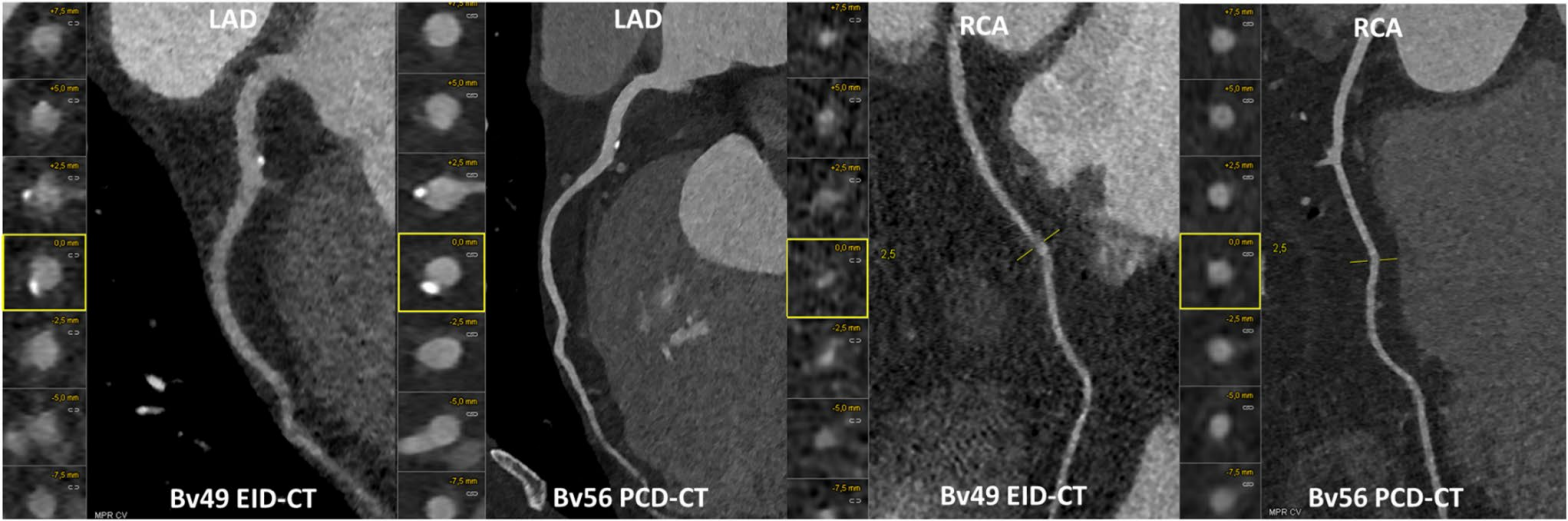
Multiplanar reconstructions of the coronary arteries of a 65-year-old post-heart transplantation patient using energy-integrating detector CT and photon-counting detector CT. On energy-integrating detector CT (EID-CT), the proximal segment of the left anterior descending artery displays a small calcification with an image quality score of 4-very good. On photon-counting detector CT (PCD-CT), the calcification is characterized as a mixed plaque (non-calcified plaque surrounding the calcification) with an image quality score of 5-excellent. For the right coronary artery, the entire artery was scored as 5-excellent with no plaque on PCD-CT. On EID-CT, the proximal right coronary artery segment was scored as 3-good with non-calcified plaque, while the mid and distal segments were rated as 2-adequate
